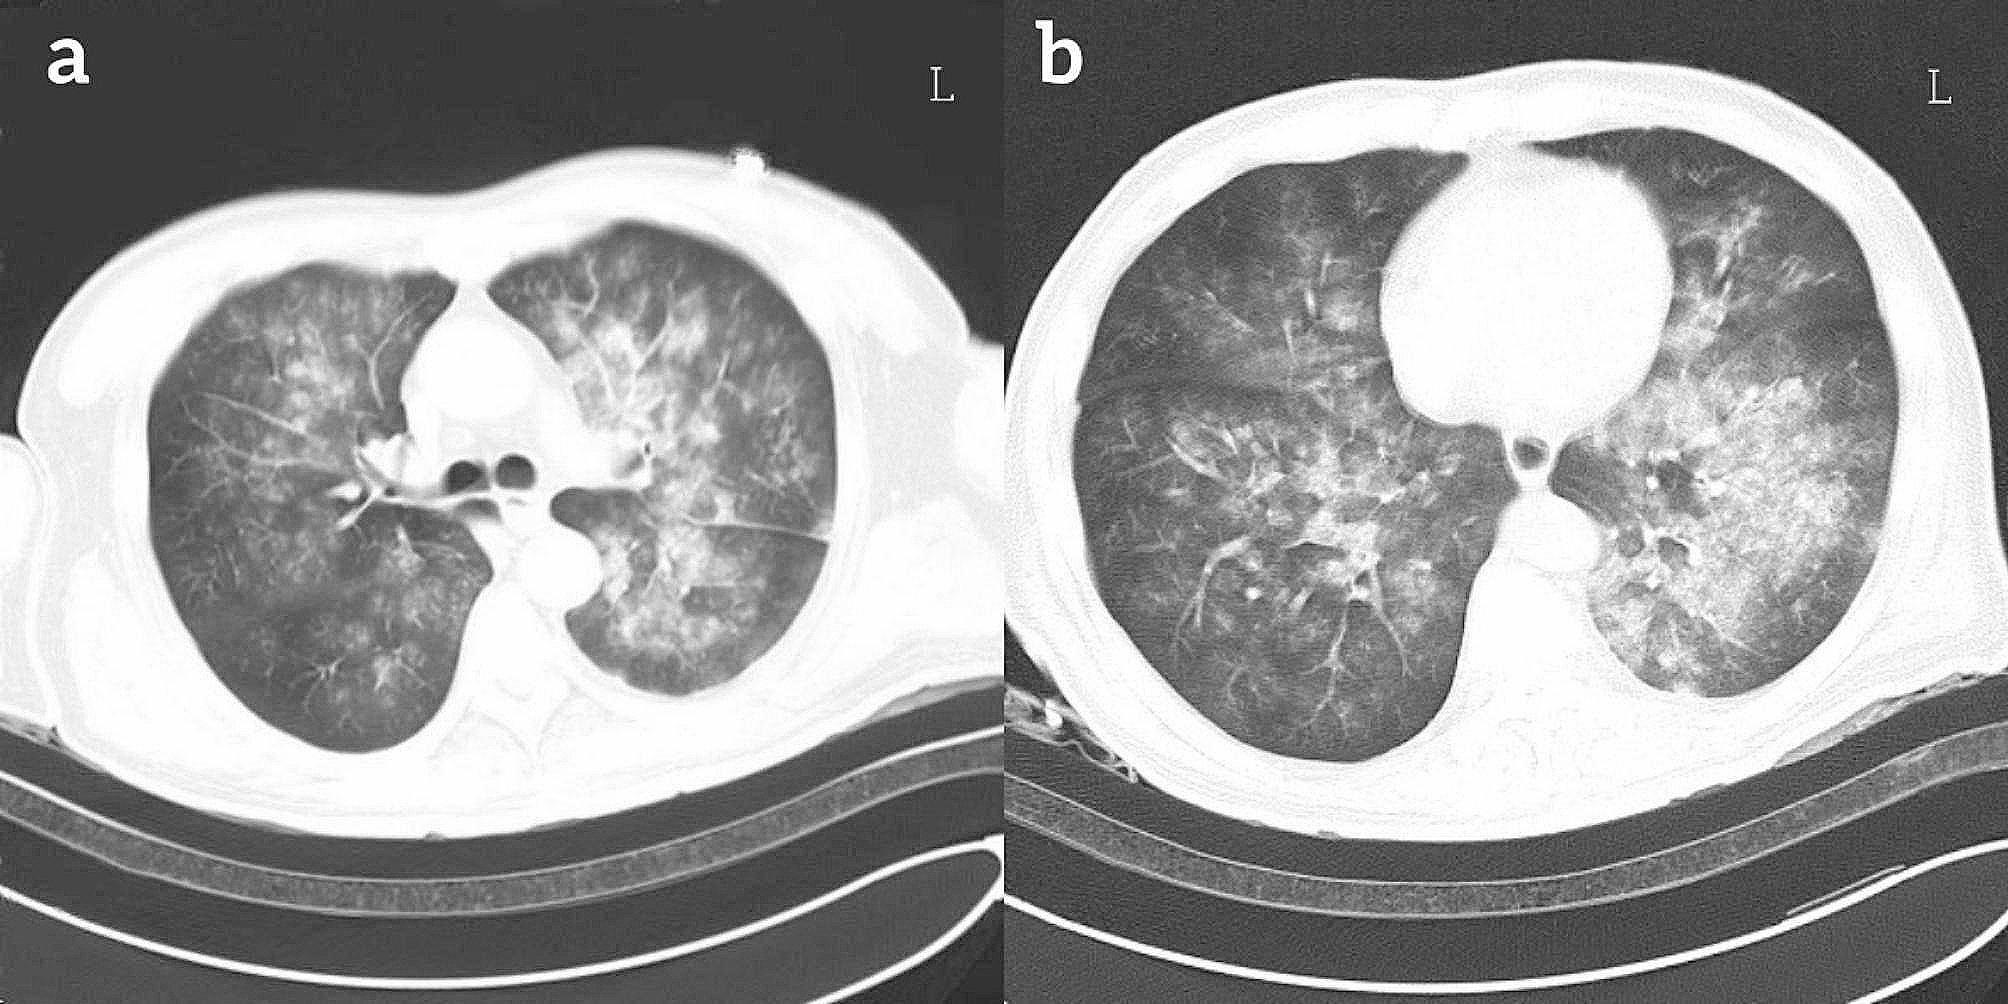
Chest computed tomography (CT) shows diffuse exudation of both lungs (a, b)
